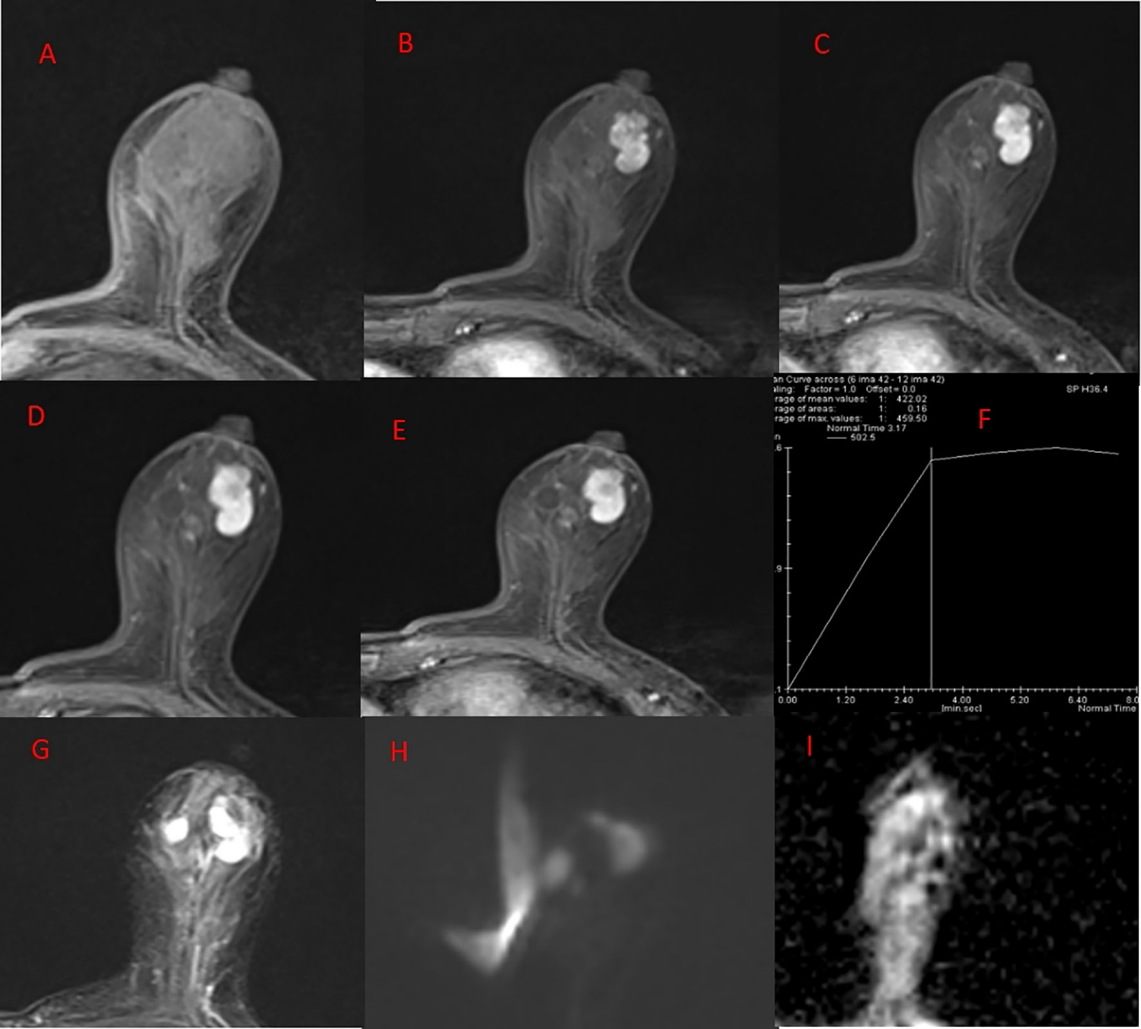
MRI plain scan + enhancement of left breast. (A) T1WI. (B–E) DCE at 1, 2, 4, 5, and 7 min, respectively. (F) TIC curve. (G) T2WI-FS. (H) DWI. (I) ADC. Left breast, outer inferior quadrant: The anterior view shows a nodular area with slightly longer T1 and T2 signal, lobulated shape, and with smooth edges and clear boundaries. DWI presents high signal intensity. ADC presents high signal intensity, with an ADC value of approximately 1.5 × 10^-3^. No significant diffusion restriction was observed. Dynamic enhancement scan shows obvious uniform enhancement, with an enhancement curve of inflow or plateau type.
